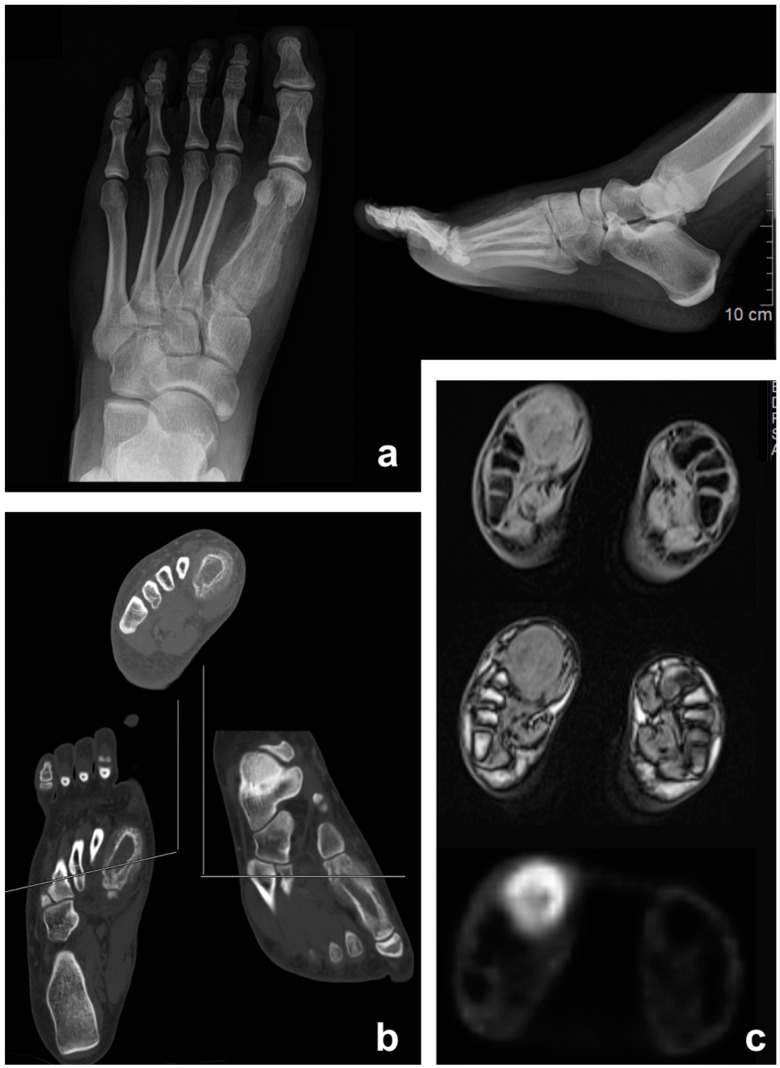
Ewing sarcoma of the foot (first metatarsal) in a 17-year-old female patient: Appearance on antero-posterior X-ray (a), CT scan (b), and PET/MRI (c).
